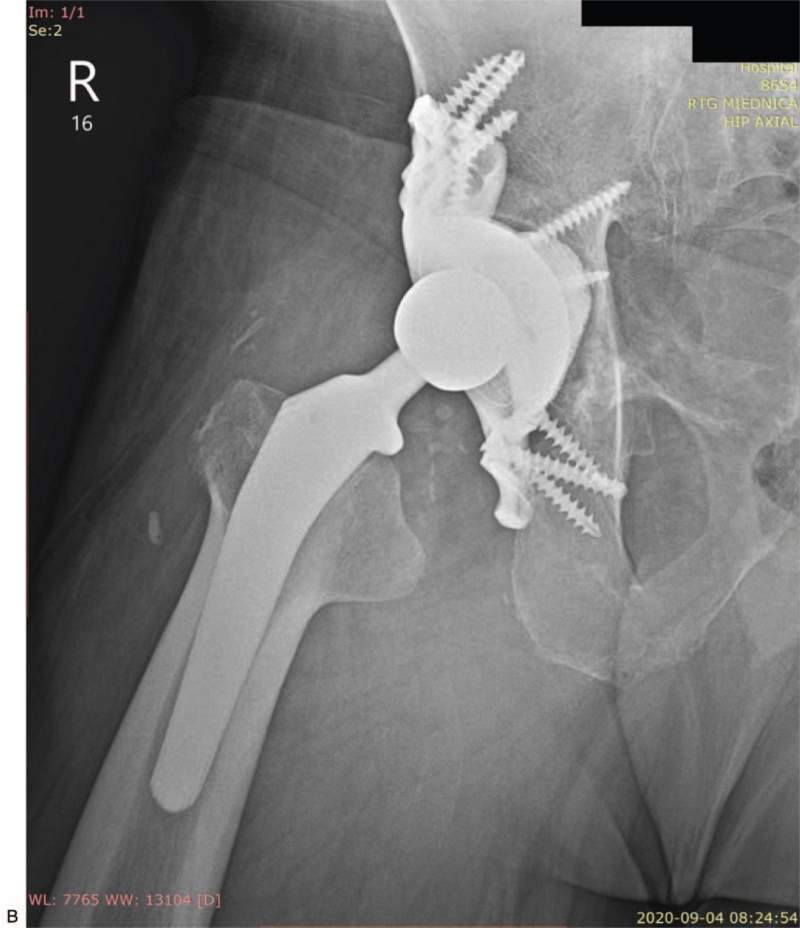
(A) X-ray of pelvis with hip joints (AP view) after revision surgery with custom-made endoprosthesis and J&J DePuy Corail head and stem on the right side, condition after left hip arthroplasty using J&J Corail method. (B) Axial X-ray of the right hip joint after revision surgery using a custom-made implant and a J&J DePuy Corail head and stem.
